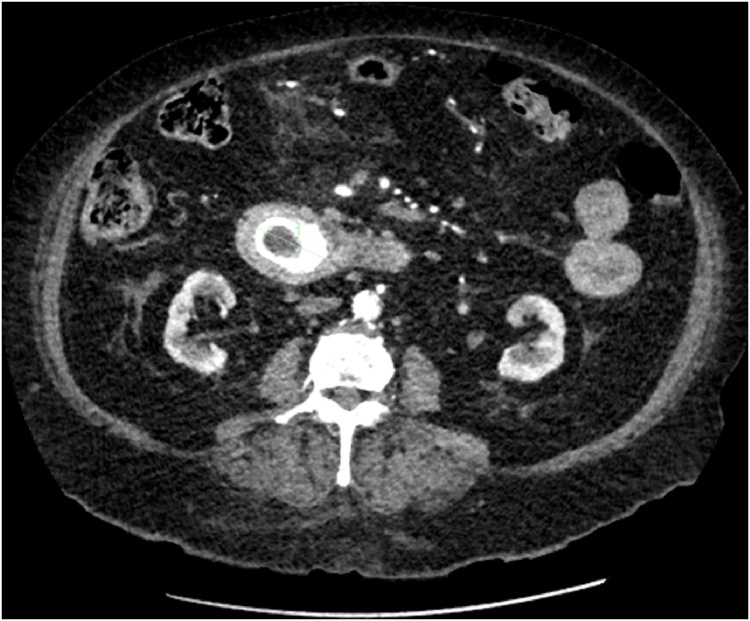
Showing 4.2 cm calcified gallstone within the duodenum on CT abdomen.
